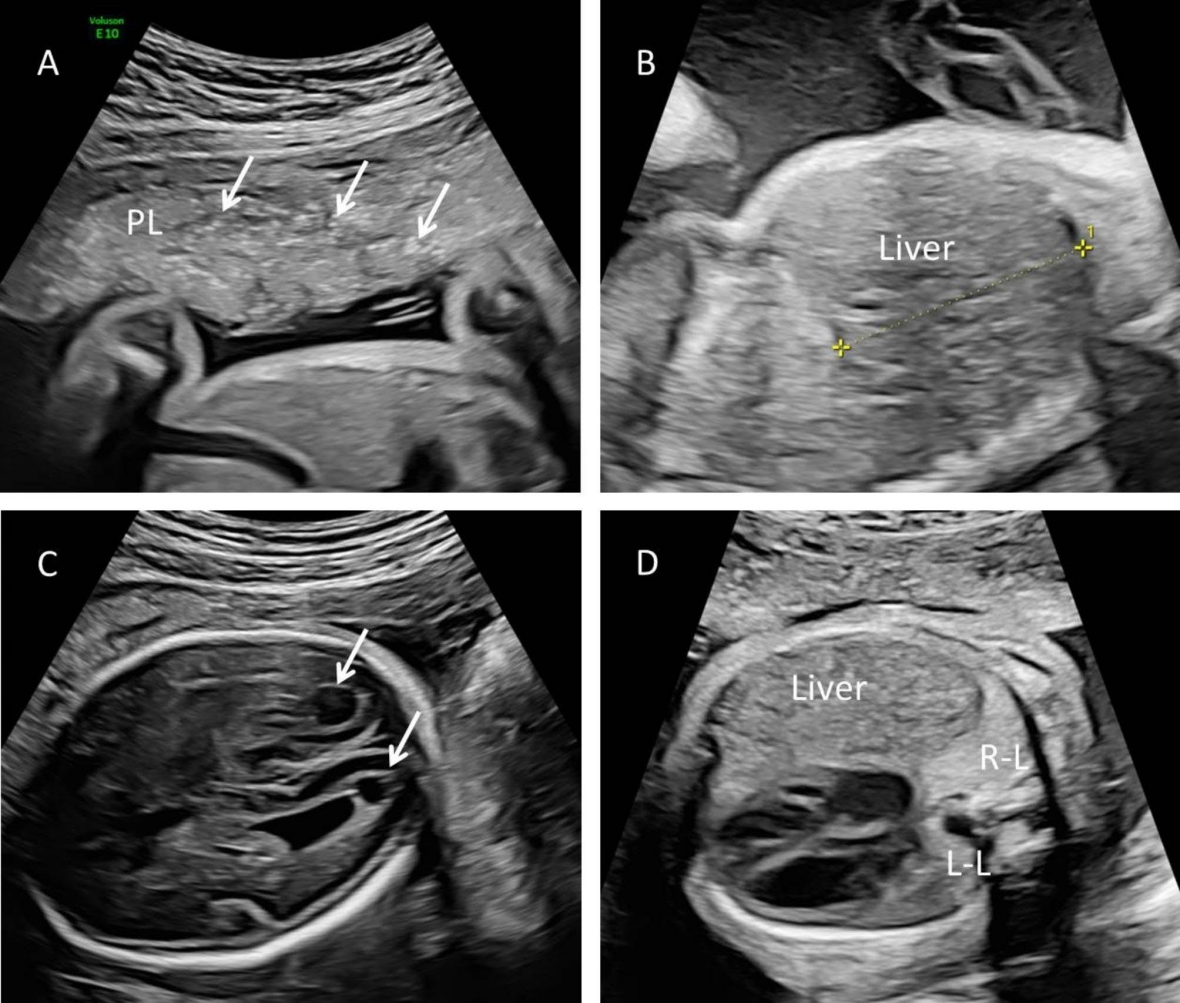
Prenatal ultrasound performed at 26^+ 3^ weeks’ gestation. (A) The placenta was diffusely distributed with punctate echogenic foci (arrows). (B) Sagittal view showed that the liver was significantly enlarged. (C) A subependymal cyst was seen in the posterior horn of the left and right lateral ventricles. (D) The transverse section of the chest showed that the bilateral lungs were significantly reduced and the echo was enhanced. PL, placenta. R-L, right lung. L-L, left lung
